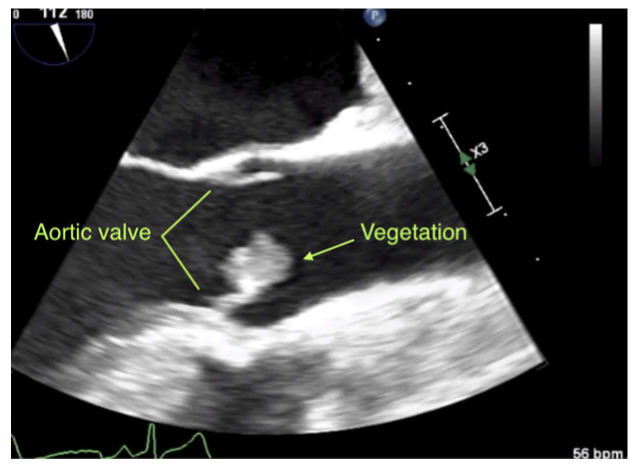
Ultrasound video (still). Transesophageal echocardiography performed in the long-axis view at midesophageal level. A visible echogenic mass, consistent with a vegetation of 12 × 12 × 10 mm^3^ adhered to the native aortic valve.
